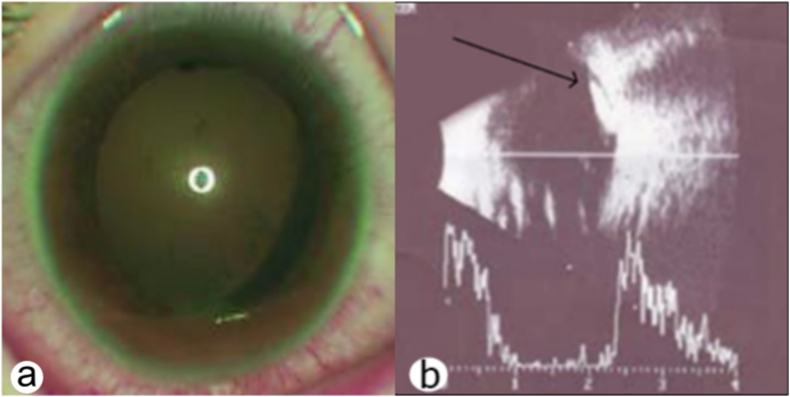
Case №1. a. Clinical presentation of internal ophthalmomyiasis. b. Ocular ultrasound demonstrating a larva (arrow).
